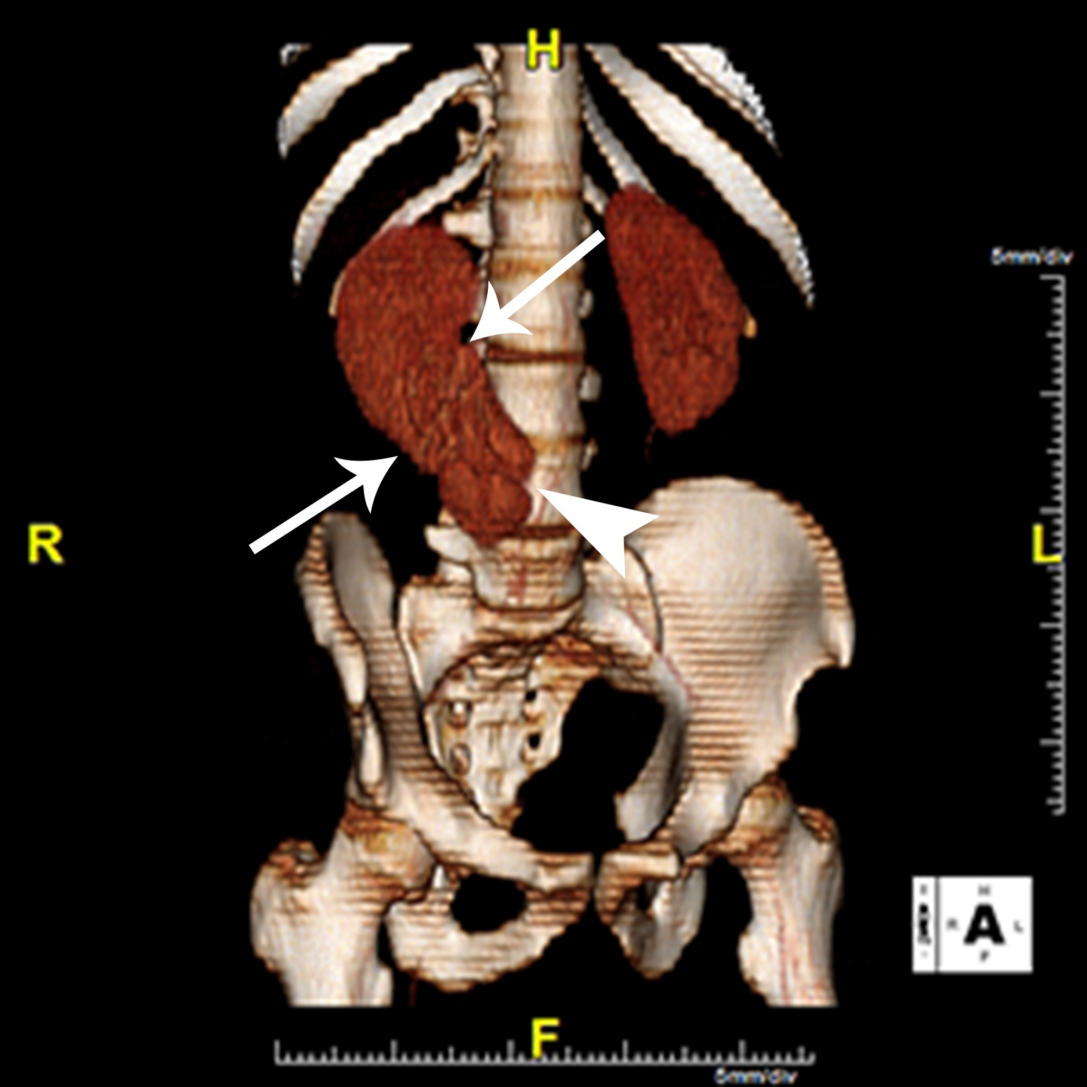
3D Computed Tomography (CT) Reconstruction Three-dimensional (3D) reconstructed CT image of the kidneys demonstrates bilateral native kidneys, as well as an ectopic supernumerary kidney fused to the lower pole of the native right kidney (white arrows). The image also demonstrates the supernumerary kidney hilum (white arrowhead).
